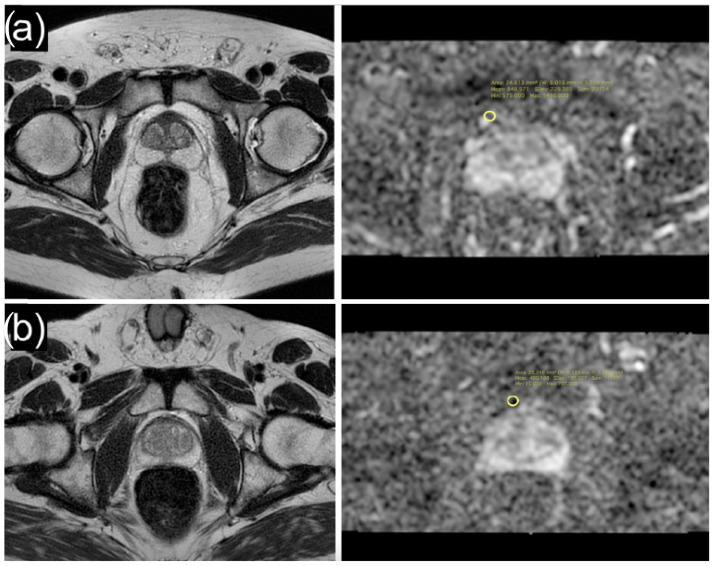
Axial T2-weighted image and ADC map of a 71-year-old patient with high ADC values (mean: 848.9 × 10^−6^ mm^2^/s) of periprostatic adipose tissue (a), and axial T2-weighted image and ADC map of a 64-year-old patient with low ADC values (mean: 405.1 × 10^−6^ mm^2^/s) of periprostatic adipose tissue (b).
